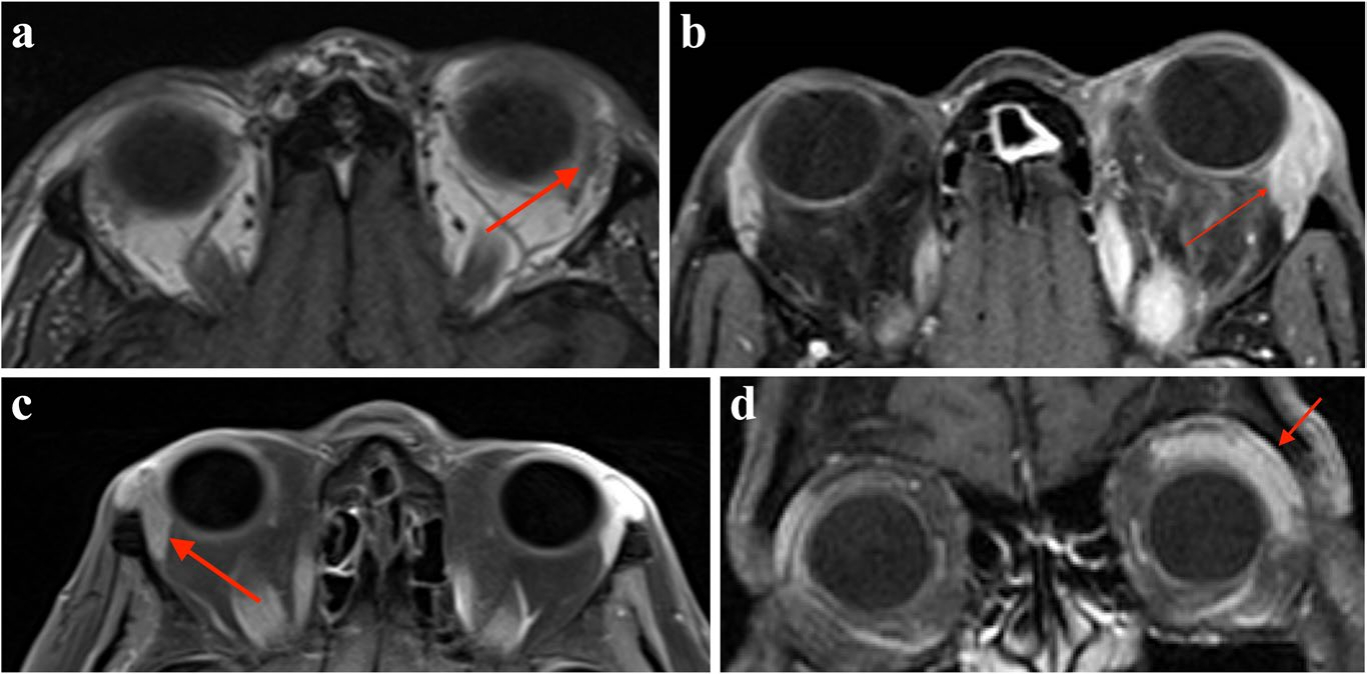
a MRI T1 image (axial view) of Case 1 demonstrating left proptosis and a marginally larger left lacrimal gland. b MRI T1 Fat suppressed post contrast image (axial view) of Case 2 demonstrating left proptosis and left lacrimal gland enlargement. c MRI T1 Fat suppressed post contrast image (axial view) of Case 3 demonstrating right lacrimal gland enlargement. 1d MRI T1 Fat suppressed post contrast image (coronal view) of Case 4 demonstrating left lacrimal gland enlargement. Arrows are included in images indicating the asymmetrical lacrimal gland enlargement
